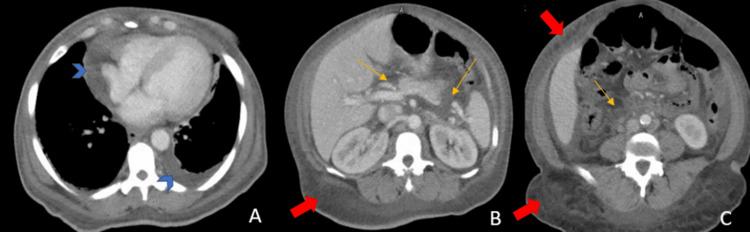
Abdominal CT result demonstrating extensive fluid overload Blue arrow head: pericardial effusion and pleural effusion, yellow arrow: abdominal ascites and periportal edema, red arrow: subcutaneous edema.
